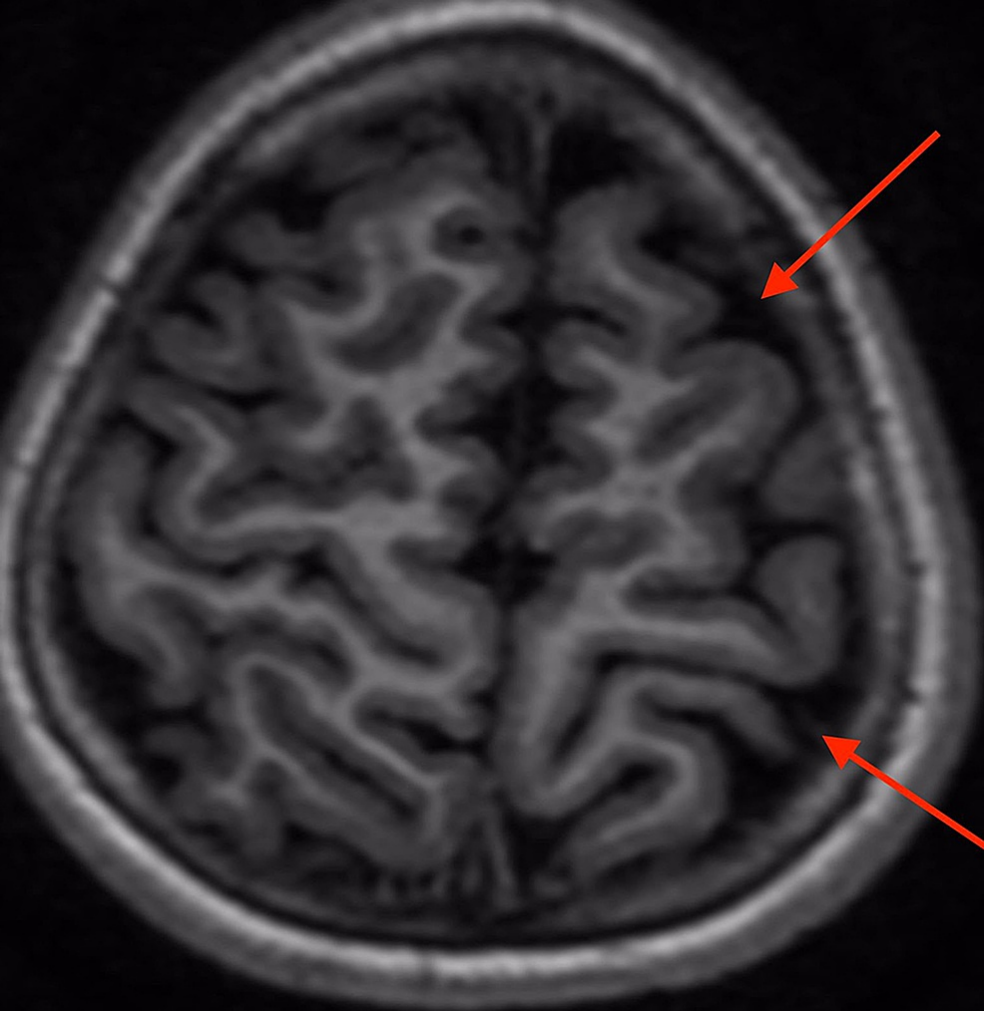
Axial T1-weighted magnetic resonance image showing subtle atrophy of the left cerebral hemisphere (red arrow). There is no cortical loss or abnormal gyral formation.
